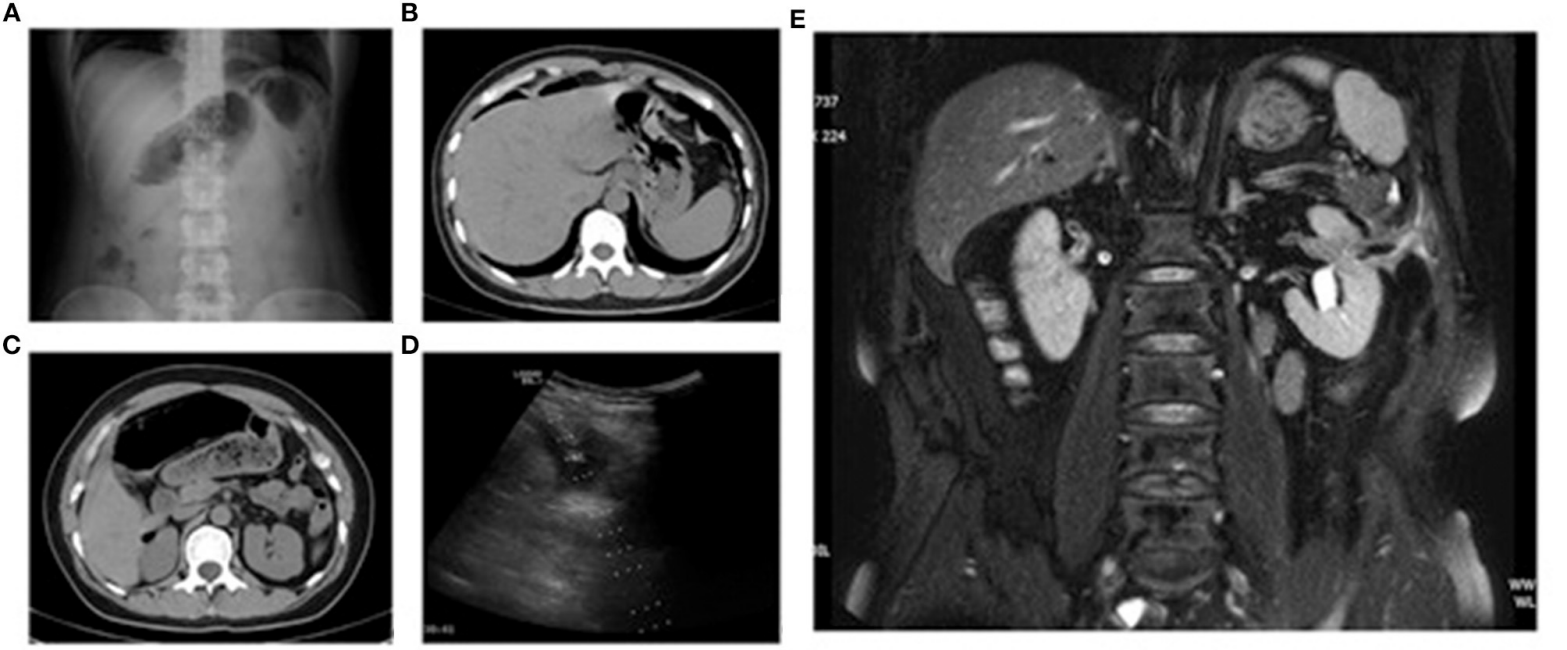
Patient number 7 (A–D): female, 40 years, myoma in the posterior wall of the uterus. Abdominal radiography (standing) and CT 1 day after microwave ablation showed free intraperitoneal air. Ultrasound showed pelvic effusion, and diagnostic paracentesis was carried out. Patient number 4 (E): male, 60 years, left renal metastasis of left-lung squamous cell carcinoma. MRI 52 days after microwave ablation showed left perirenal abscess and fistula between the intestines, ablation area, and urinary system. (A) Radiography of the abdomen (standing) 1 day after microwave ablation. (B) CT 1 day after microwave ablation. (C) CT 1 day after microwave ablation. (D) Ultrasound 1 day after microwave ablation. (E) MRI 52 days after microwave ablation.
